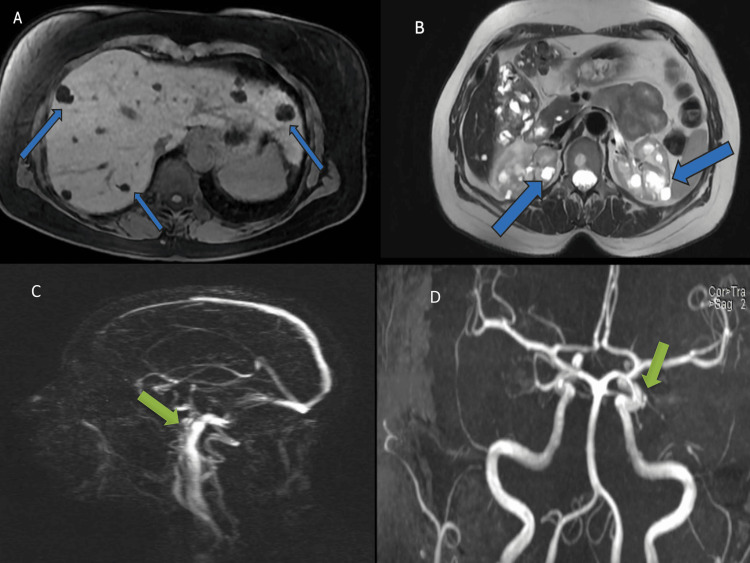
Abdominal magnetic resonance imaging (MRI) (A, B) and cerebral magnetic angiography (MRA) (C, D). Abdominal MRI revealed multiple bilobar hepatic cysts (A) and numerous bilateral sizeable renal cysts, some of them with hemorrhagic content, located predominantly in the renal cortex (B). Hepatic and renal cysts are pointed out with blue arrows. Cerebral MRA showed an aneurysm of the right internal carotid artery, in close proximity to the ophthalmic artery, measuring at 3.6 x 2.6 x 3.5 mm as pointed out with green arrows (C, D).
